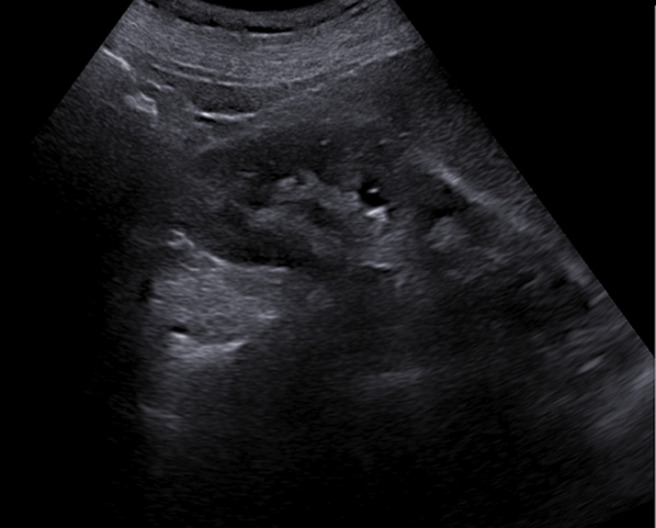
postoperative ultrasound imaging of the right kidney
